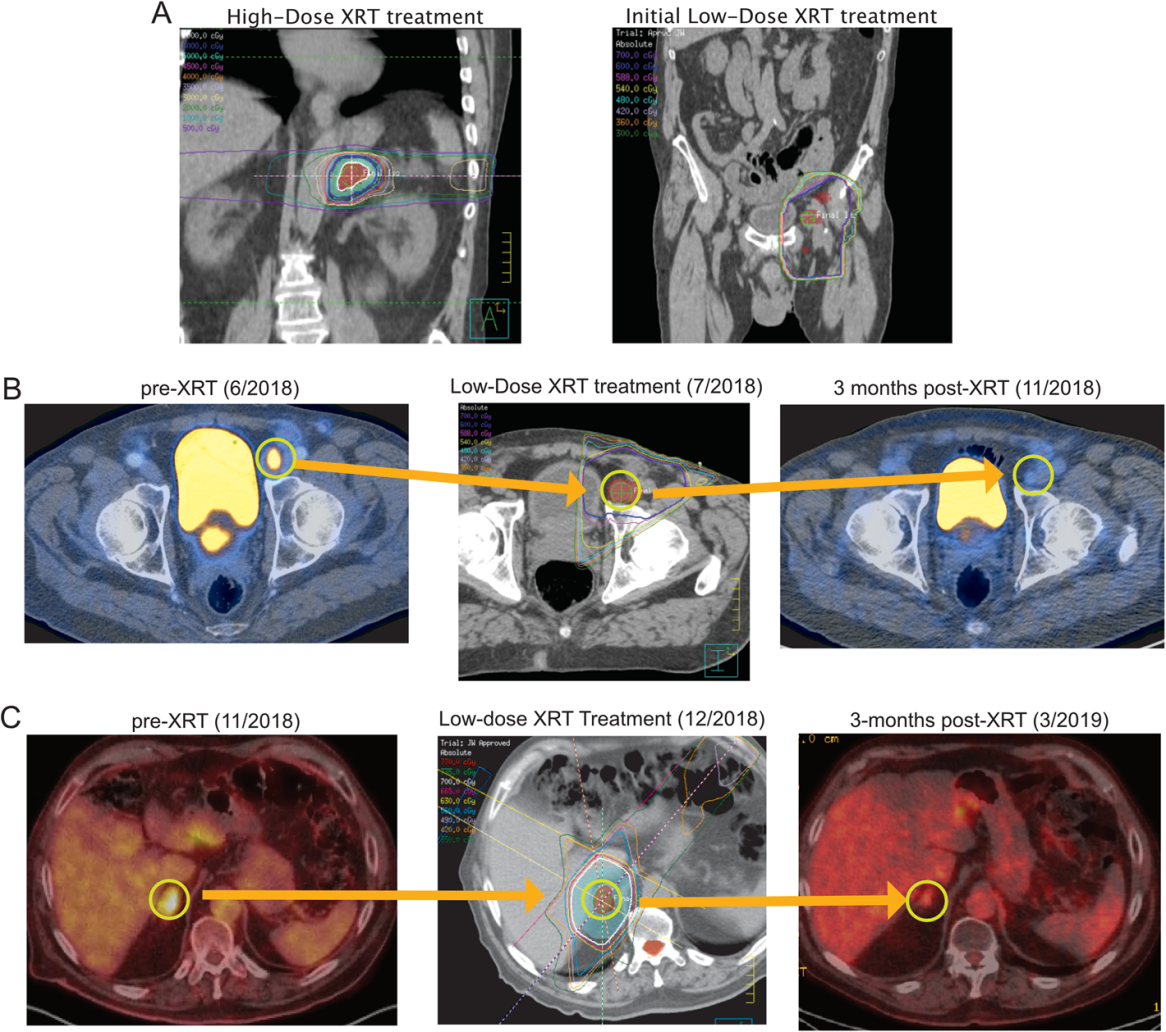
Representative scans from a patient receiving intentional low-dose radiation. Scans from a 69-year-old patient with Merkel cell carcinoma with previous disease progression on atezolizumab and bevacizumab who was given low-dose radiation to an involved inguinal node. An area receiving no radiation in the right adrenal gland developed a metastasis 3 months later, which was subsequently treated and shown to have improved radiographically as well
